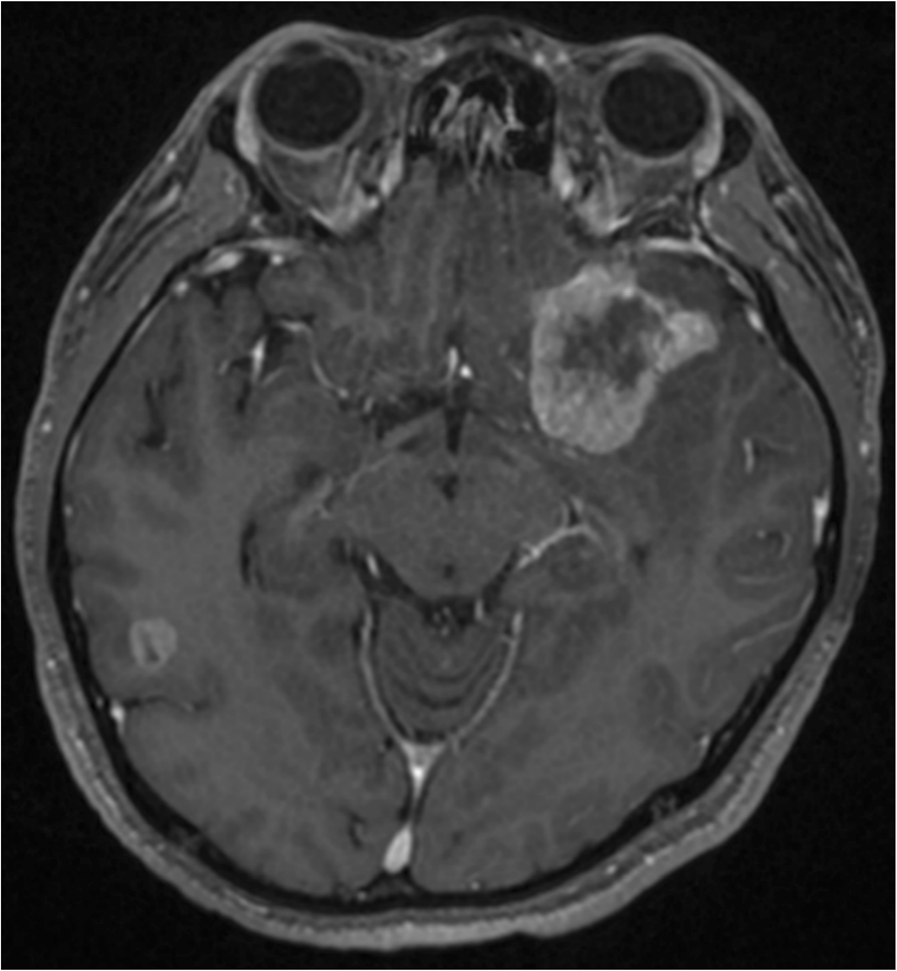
Brain magnetic resonance imaging at first visit to our hospital
